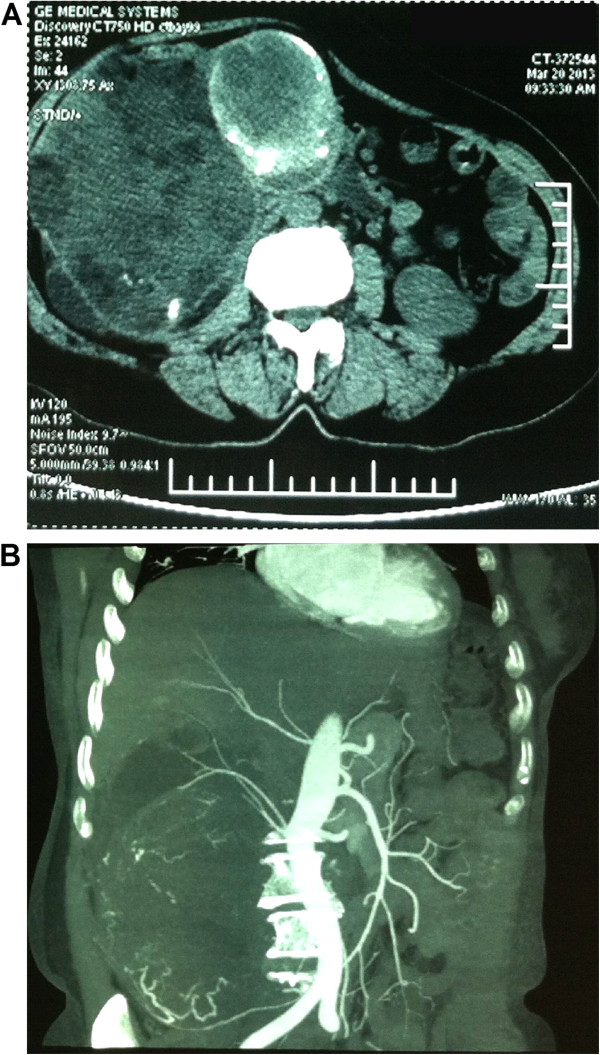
Computed topography (CT) of the abdomen. A, CT scan images showed a huge lobulated cystic-solid tumor occupying the perirenal space of right kidney. B, The coronal reconstruction image showed a shadows of multiple tortuous vessels at the margin of huge mass.
